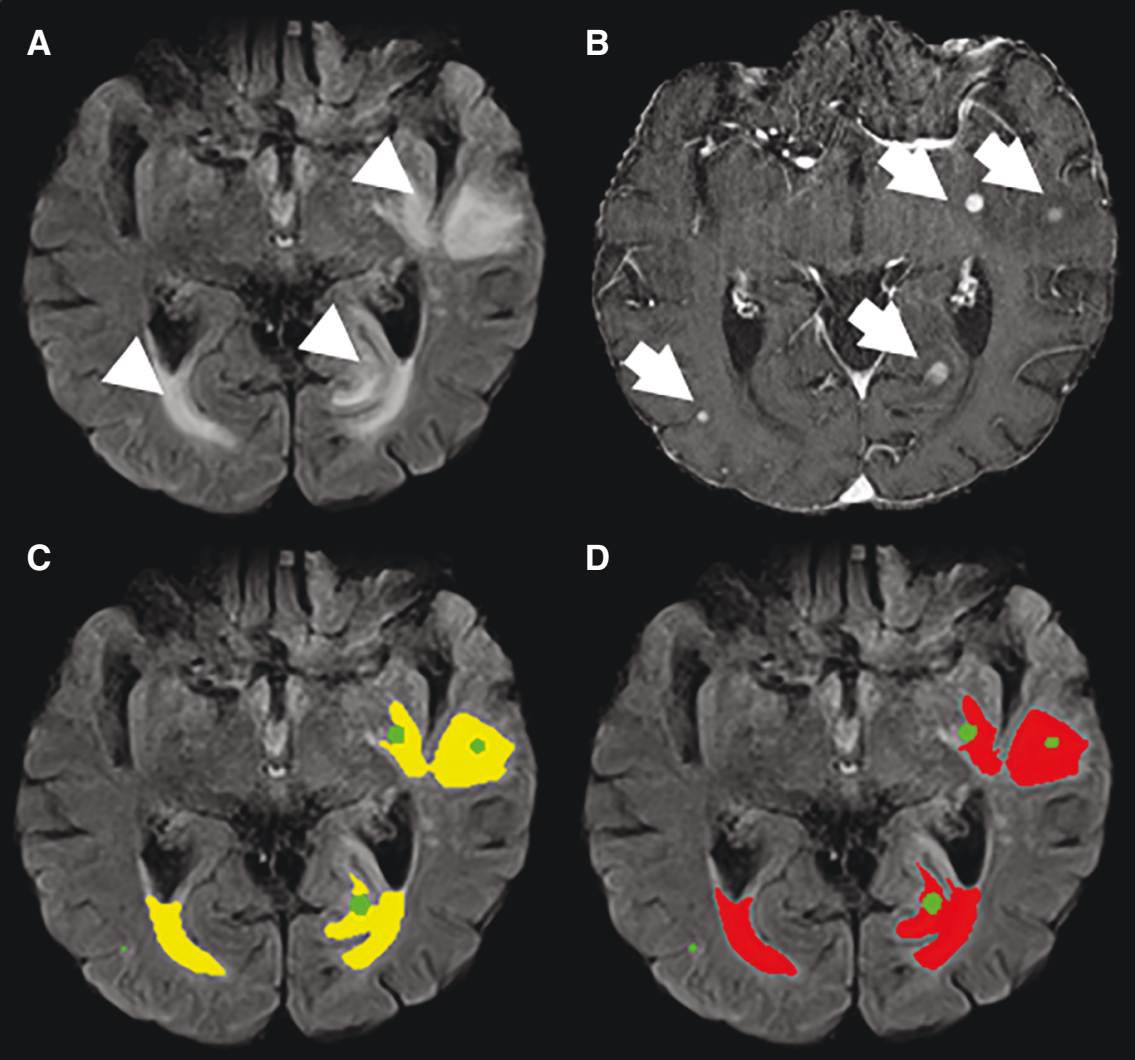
Example of an MRI study with axial FLAIR and T1-weighted post-contrast images of a 71-year-old male patient with malignant melanoma and multiple BM in the institutional dataset (B, arrows and green) and perifocal edema (A, arrowhead). Our HD-BM algorithm detects the perifocal edema accurately (C, yellow) compared to the ground-truth segmentation (D, red).
